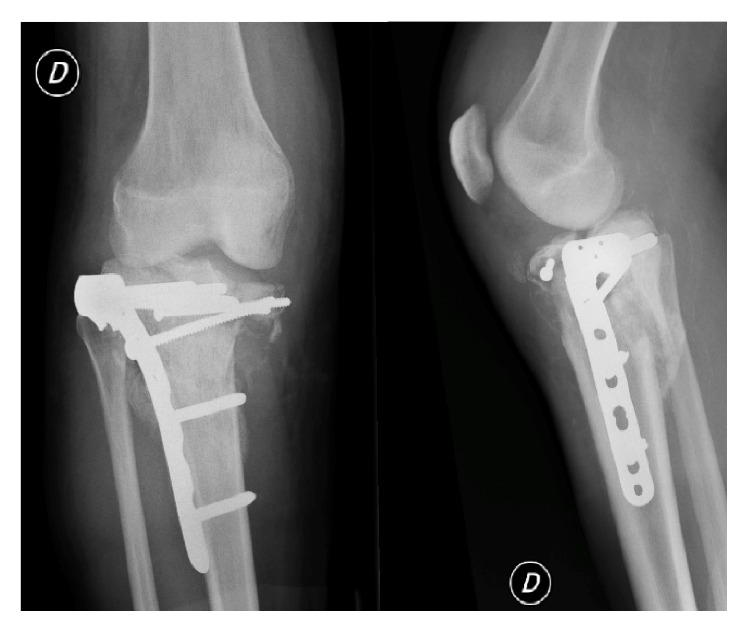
Follow-up X-rays at three months postoperatively of the right knee.
